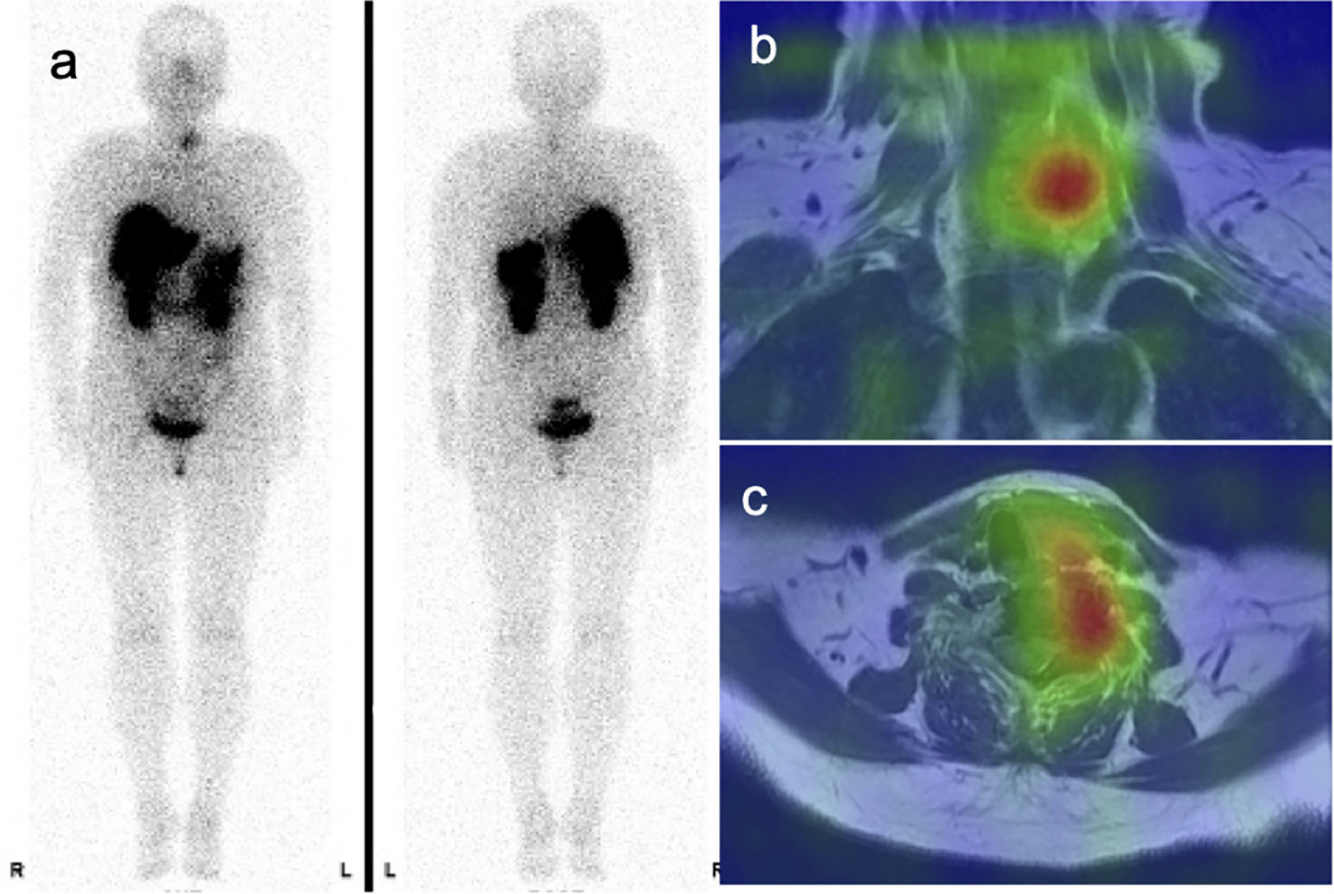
Diagnostic imaging findings. Somatostatin receptor scintigraphy with high sensitivity for diagnosing tumor-induced osteomalacia and detecting a positive lesion found radiotracer uptake in the ventral aspect of the C7–T1 vertebrae (a–c).
